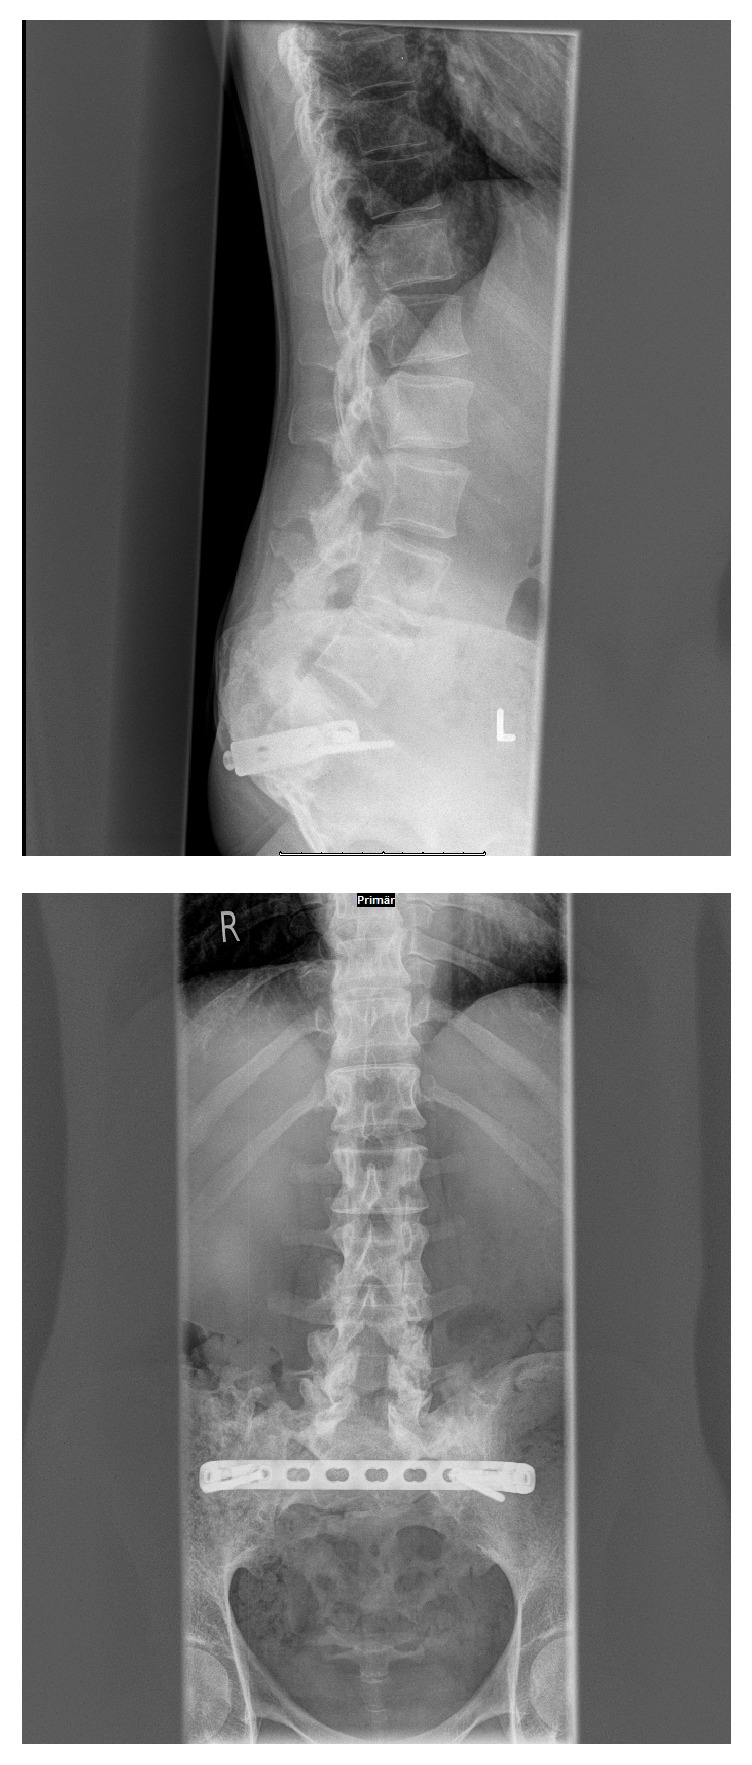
X-ray of the lower spine with ilioiliacal plate.
